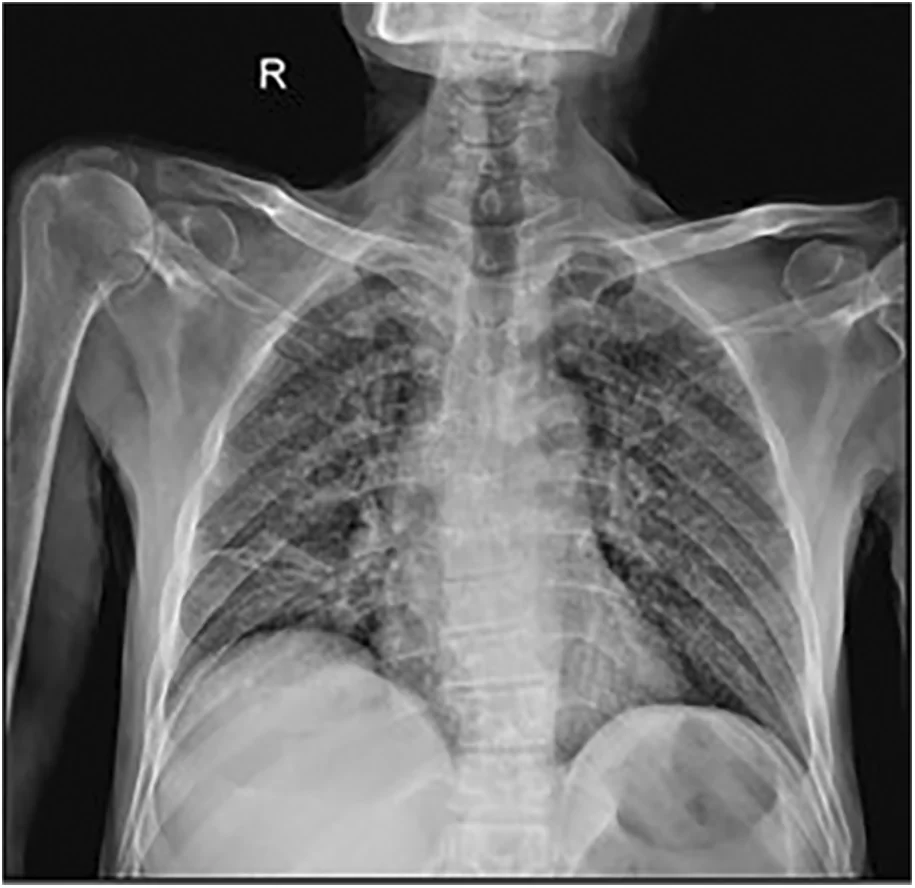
Chest radiograph obtained on the first day of admission demonstrating bilateral diffuse patchy reticulonodular opacities, predominantly involving the upper lobes and perihilar regions.
